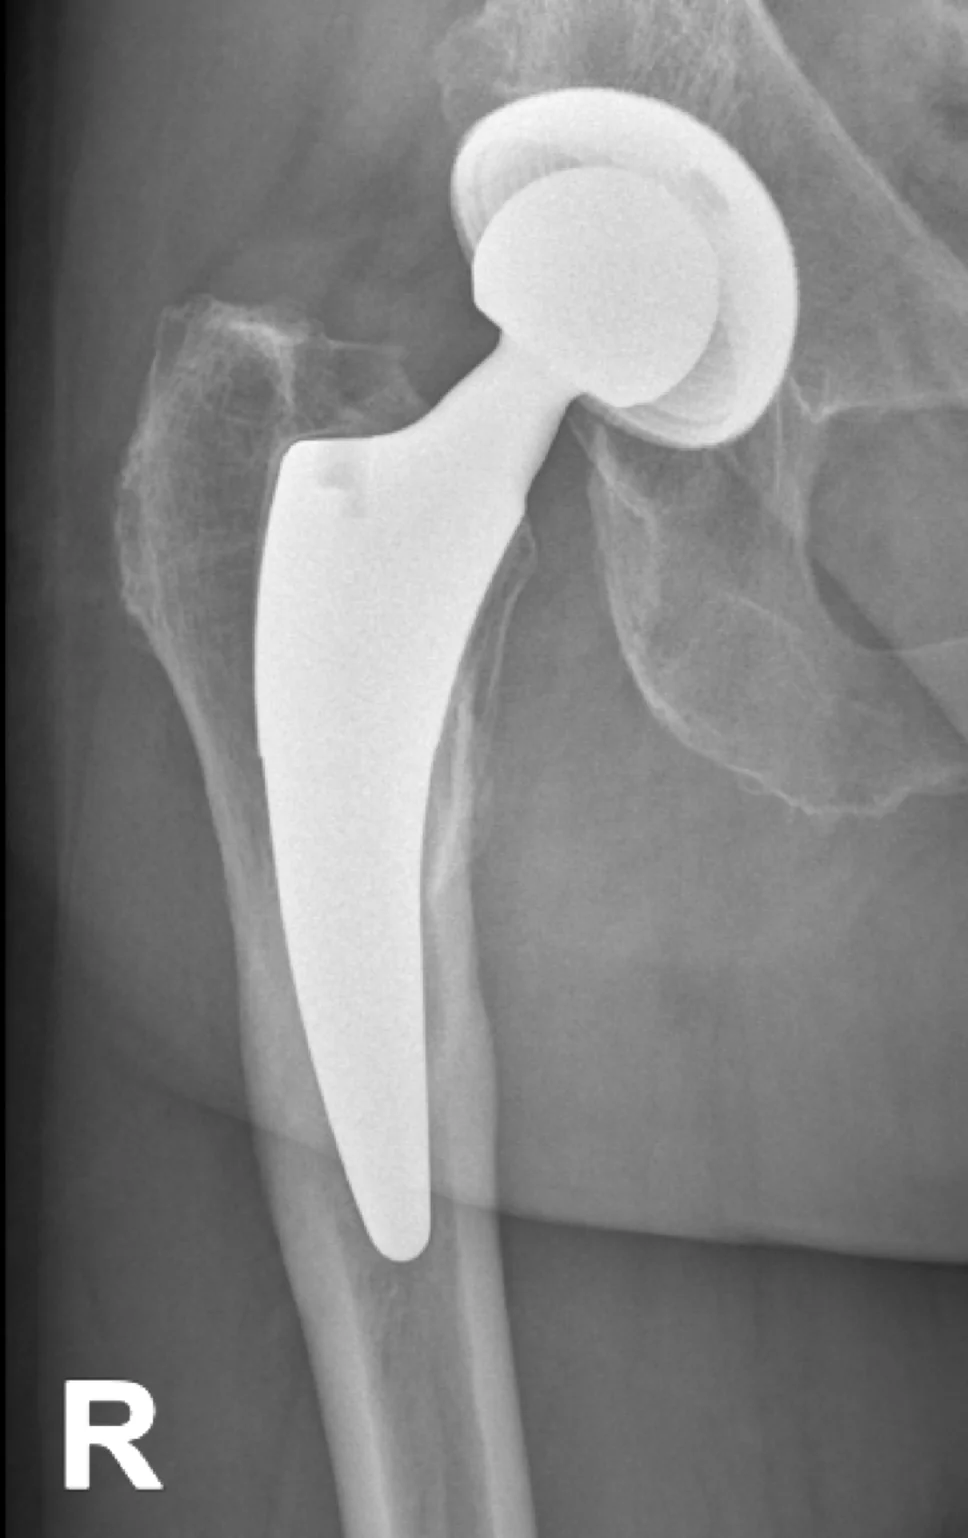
Radiographs of a 67 year old female patient with a short femoral Fitmore stem. Cortical hyperthrophies in Gruen Zone 3, 5 and 6 can be observed
